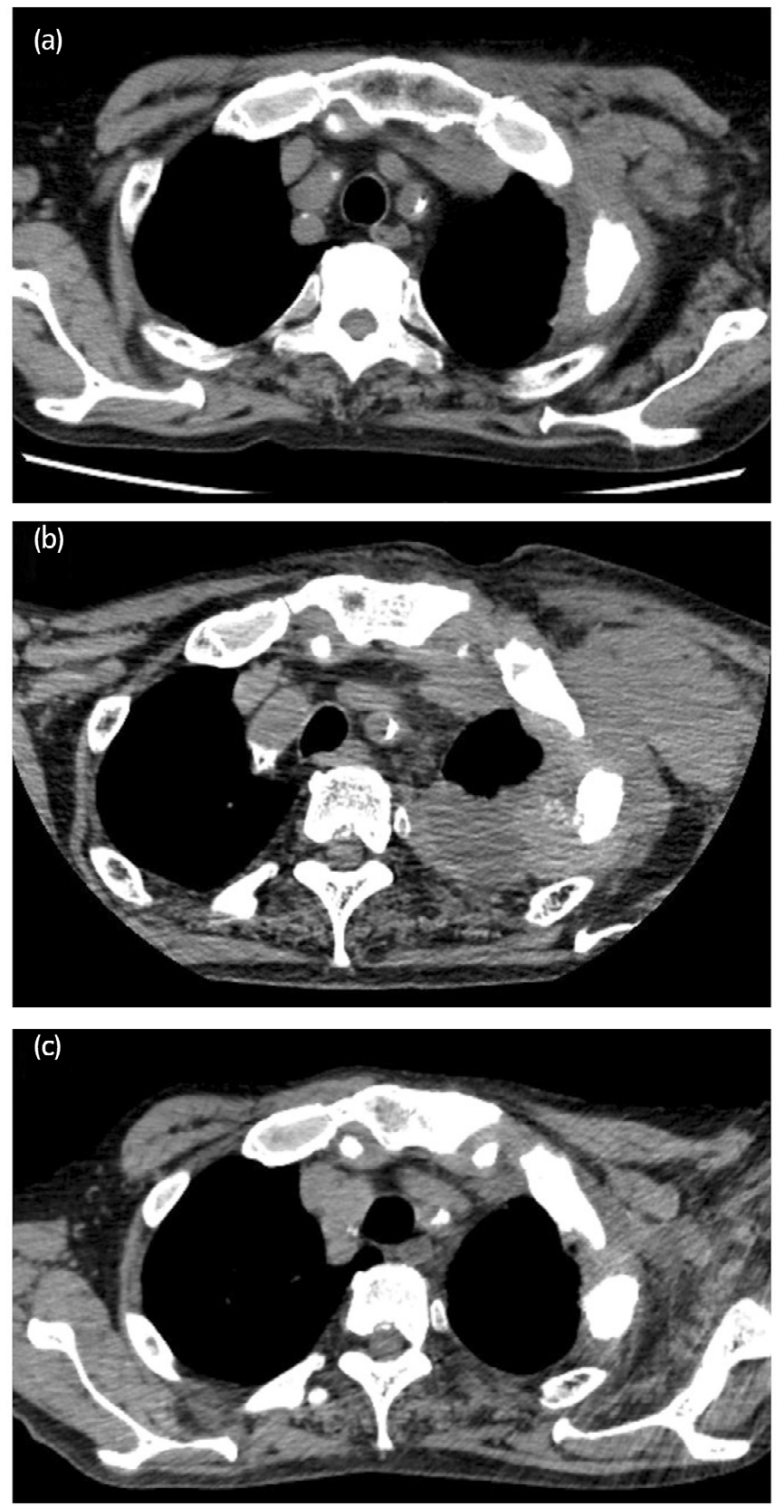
Tumor response to pembrolizumab. (a) Computed tomography showed a swelling of the left axillary lymph node, measuring 46.6 × 23.3 mm, before PBLZ administration. (b) After four cycles of PBLZ, the left axillary lymph nodes were severely enlarged, measuring 74.6 × 39.5 mm (an increase of 160% from baseline tumor burden), and malignant left pleural effusion was observed. (c) Five months after the final dose, the left lymph node adenopathy, and the pleural effusion were dramatically diminished.
